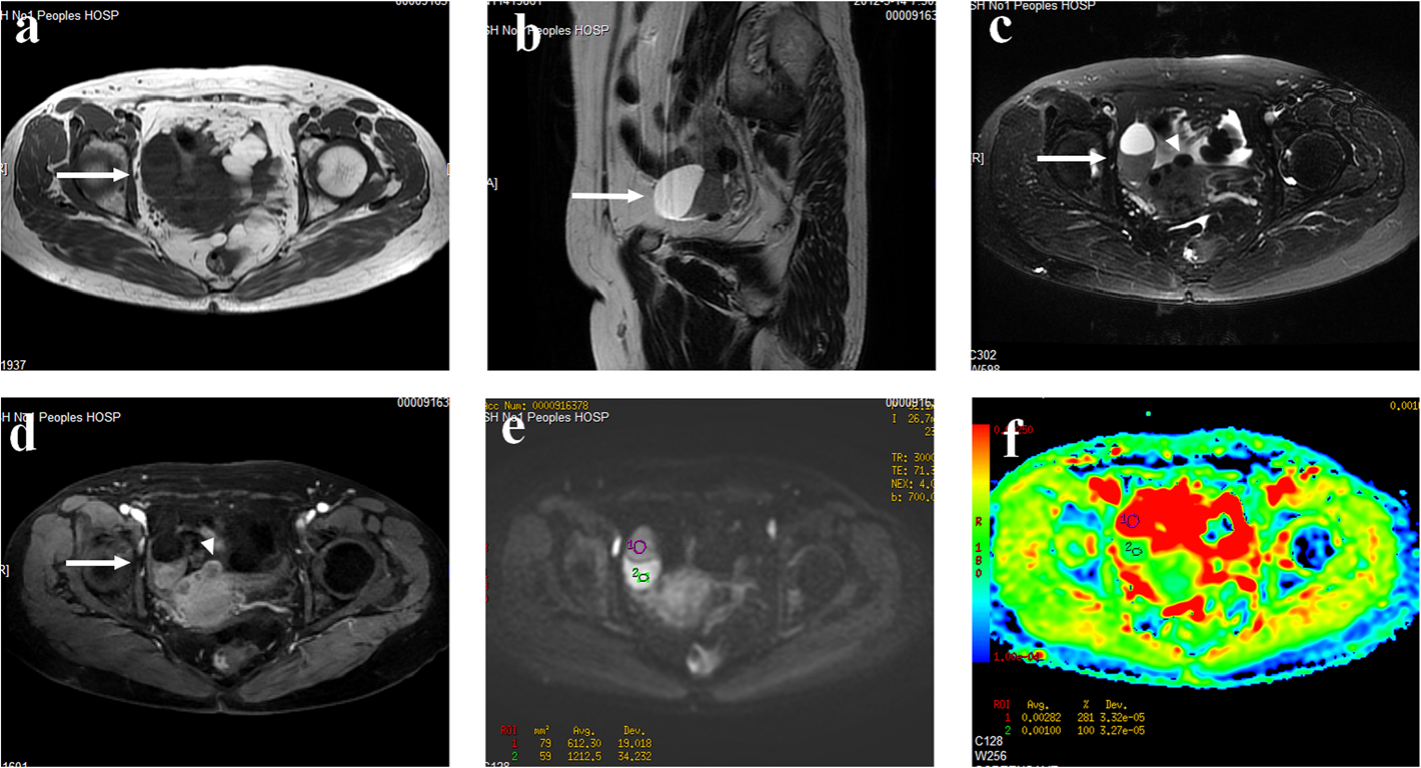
A 60-year-old female patient with luteinized thecoma. (a) Axial T_1_WI revealed a cystic solid mass with hypo–isointense signal in the right adnexal region (arrow). (b) On sagittal T_2_WI, the cystic component of the tumor gave a homogeneous hyperintense signal. Note that degenerated uterine fibroids (arrowhead) were also detected. (c) On fat-suppressed T_2_WI, the signal of the mass was similar to that in (b). (d) On contrast-enhanced fat-suppressed T_1_WI, the solid components (arrow) of the lesion showed moderate enhancement, like that of the myometrium. (e) On DWI-MRI (*b* = 700 s/mm^2^), the upper part of lesion appears as a homogeneous isointense signal and the lower part as a hyperintense signal. (f) ADC map shows the marked hyperintense signal of the cystic components (T2 shine-through effect) and the isointense signal of the solid components. The ADC values at the corresponding sites are 2.82 × 10^–3^ mm^2^/s (cyst) and 1.00 × 10^–3^ mm^2^/s (solid).
